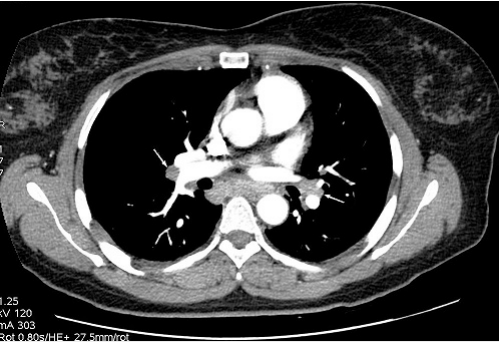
Cardiac magnetic resonance imaging (MRI) with gadolinium contrast: evidence of delayed hyper enhancement (arrow) in both the basal and inferolateral left ventricular regions
